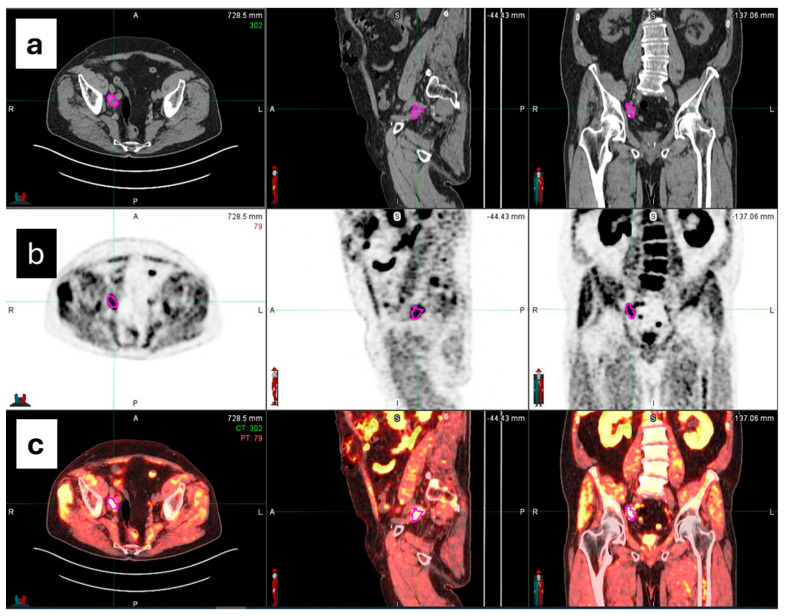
The manual segmentation of volumetric regions of interest (VOIs) on PET/CT images (c) around the area of focal ^18^F-choline uptake using MiM Maestro software. Each contour (pink line) was superimposed on CT (a) and PET (b) images.
